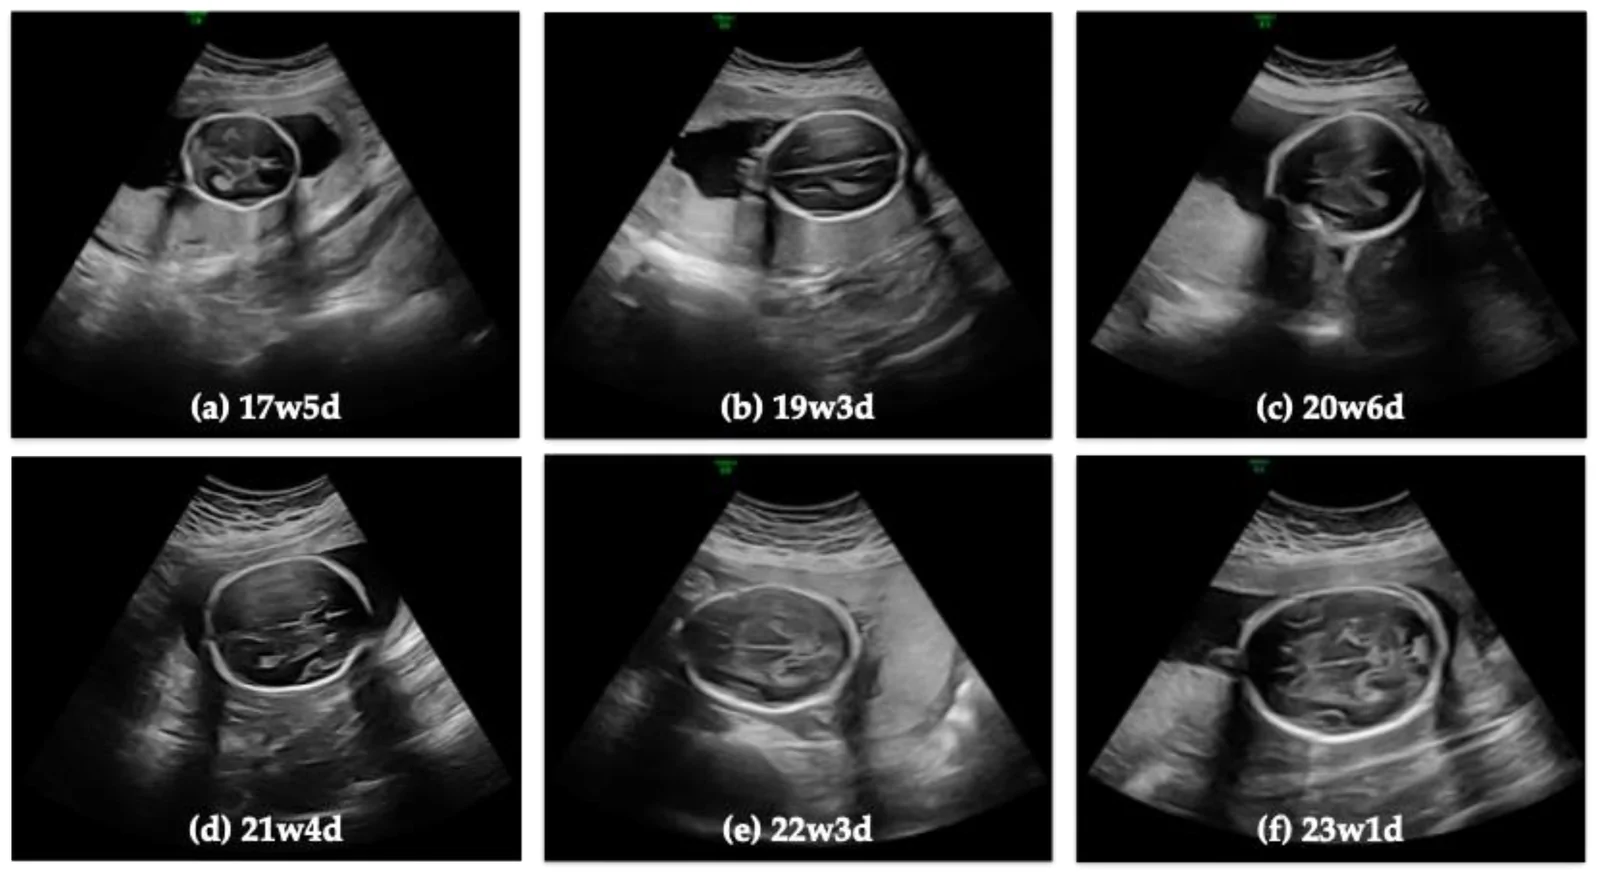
Examples of standard fetal head ultrasound images from the locally acquired clinical dataset across different GAs. Examples obtained from six different pregnancies are shown, corresponding to gestational ages of (a) 17w5d, (b) 19w3d, (c) 20w6d, (d) 21w4d, (e) 22w3d, and (f) 23w1d. All images represent standard transthalamic planes used for routine fetal head biometry and demonstrate the variability in fetal anatomy and ultrasound image characteristics across gestation.
